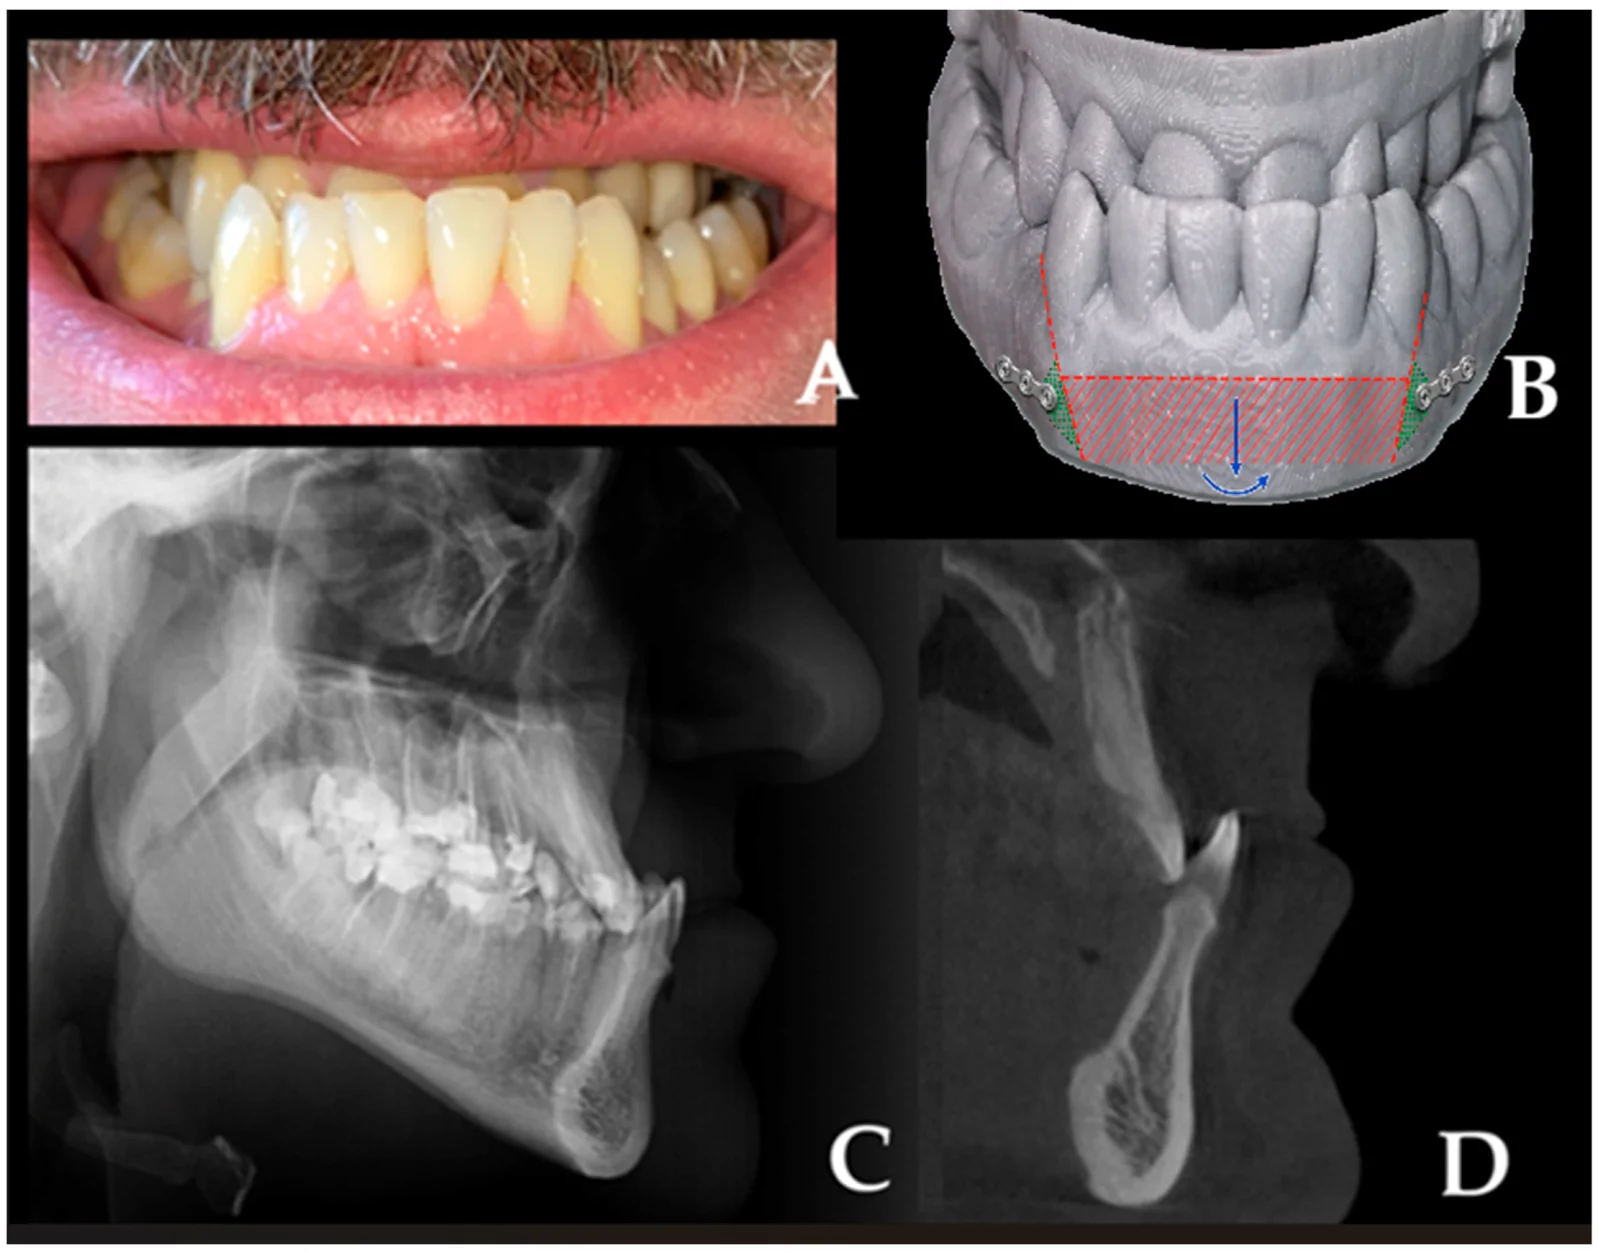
There are many types of dental malocclusion that can be easily treated with some basic orthodontic appliances. The scope of each skeletal malocclusion, on the other hand, quite commonly requires a collaboration between maxillofacial surgeons and orthodontists. Etiology, severity, and oral, dental, and facial characteristics in each case might have some typical or atypical features. The disproportions in bone shape, volume, position, and three-dimensional relations affect the scope of possible approaches and levels of the scheduled treatment plan. Most commonly, typical osteotomies used in orthognathic surgery (OS) are related to the scope of maxillary bone osteotomy according to the Le Fort I principle, as well as bilateral sagittal split osteotomy (BSSO) of the mandible [1,2,3]. Each osteotomy has its own modifications, alterations, and other specially designed scopes of surgical cuts, osteotomies, ostectomies, or others. Because of recent advances in three-dimensional cone-beam computed tomography (CBCT) as well as individual patient solutions (IPSs) and virtual planning, great improvements in the scope of planning and processing with surgeries are known. In complex segmental deformities, CBCT-based analysis may help distinguish whether the discrepancy is generalized skeletal malposition or a localized dentoalveolar problem requiring a more selective osteotomy design. Each OS not only affects bite, skeletal relation, and facial esthetics, but also function and stability of TMJ (temporomandibular joints), as well as other aspects, like the positive effect on airways, decreasing snoring, and OSAS (obstructive sleep apnea syndrome) symptoms [3]. Nowadays, some atypical and “old-school-based” osteotomies are not that common; however, they can greatly affect patients’ outcomes and final results if they are planned based on a novel virtual, CBCT-based IPS protocol. Mandibular segmental osteotomies may involve anterior, lateral, or total dentoalveolar segments depending on the location and extent of the deformity [1,2,3,4,5]. The anterior mandibular procedure has historically also been termed “subapical mandibular osteotomy,” “anterior subapical osteotomy,” or the “Köle procedure”; for consistency, the term “mandibular anterior segmental subapical osteotomy (MASSO)” is used throughout this report. The following radiological images represent the situation when the upper incisor crown is almost entirely covered by the lower incisors, while both lateral segments remain on the same level in both clinical and 3D-printed model evaluation (A,B); the red line marks the scope of anterior segment elevation in the presented skeletal malocclusion that requires osteotomy and its down-positioning, marked as red–green markings. This finding is important because it suggests that the main vertical discrepancy is concentrated in the anterior mandibular dentoalveolar segment, whereas the lateral mandibular segments preserve a more acceptable occlusal level. On the other hand, very valuable information is addressed by a routine cephalometric radiograph and CBCT in the sagittal plane in order to assess the scope of occlusal plane tilting, dentoalveolar relations, and the scope of anterior mandibular tooth-segment elevation towards the lateral mandibular aspects (C,D). Therefore, CBCT was useful not only for diagnosis and planning but also for understanding the biological limits of movement and the risk of postoperative periodontal complications associated with this uncommon reversed Köle-type MASSO.
